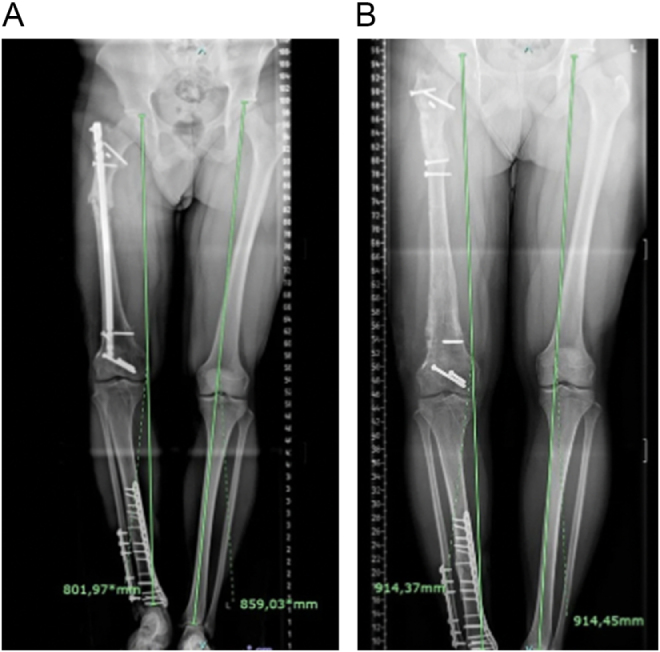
(A) Pre-operative full leg radiograph, (B) Final full leg radiograph after removal of external fixator at18 months after first surgery (residual varus mechanical alignment is present).
